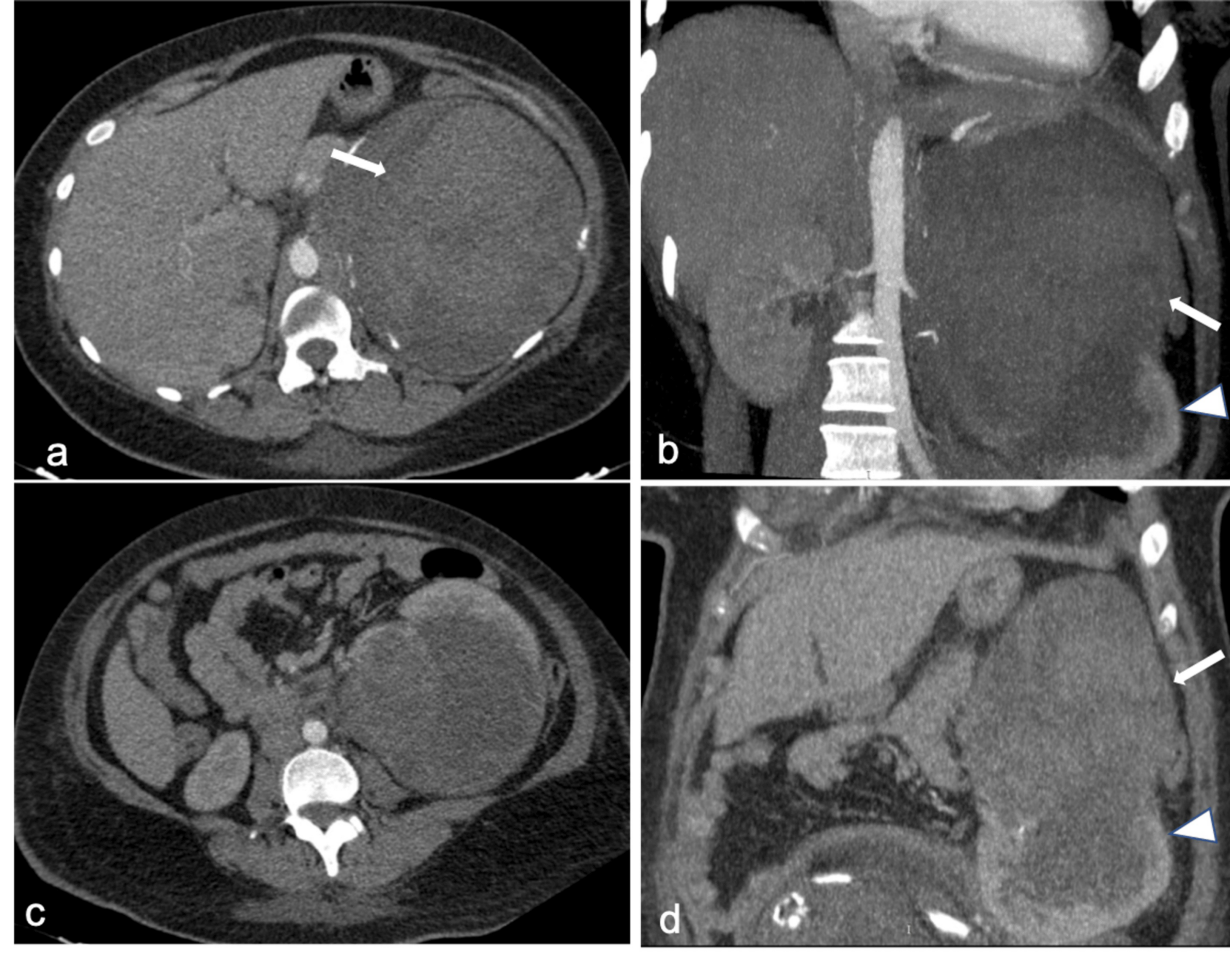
CT findings. (a, b) Axial and coronal arterial maximum intensity projection (MIP) images show heterogeneous enhancement with a predominant hyperdense component with minimal hypervascularity along the upper pole of the left kidney (arrow). (c, d) Axial and oblique coronal MIP images in the portal venous phase show similar findings with no obvious active bleeder or pseudoaneurysm. Normal enhancing lower pole of the left kidney can be noted (arrowhead).
